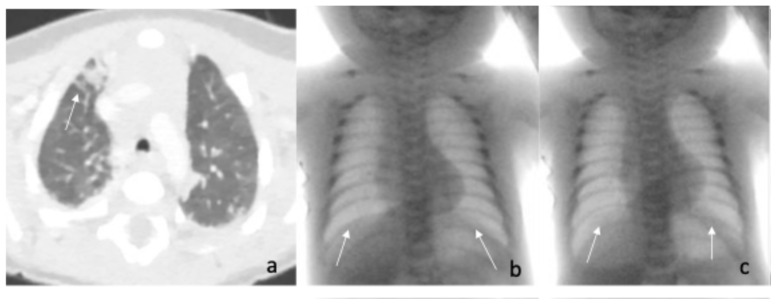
(a): Computed tomography angiography of the pulmonary arteries: the white arrow indicates an area of consolidation. (b,c): Expiratory (on the left side) and inspiratory (on the right side) fluoroscopy sequences. The white arrows show the poor diaphragmatic excursion in the two respiratory phases.
